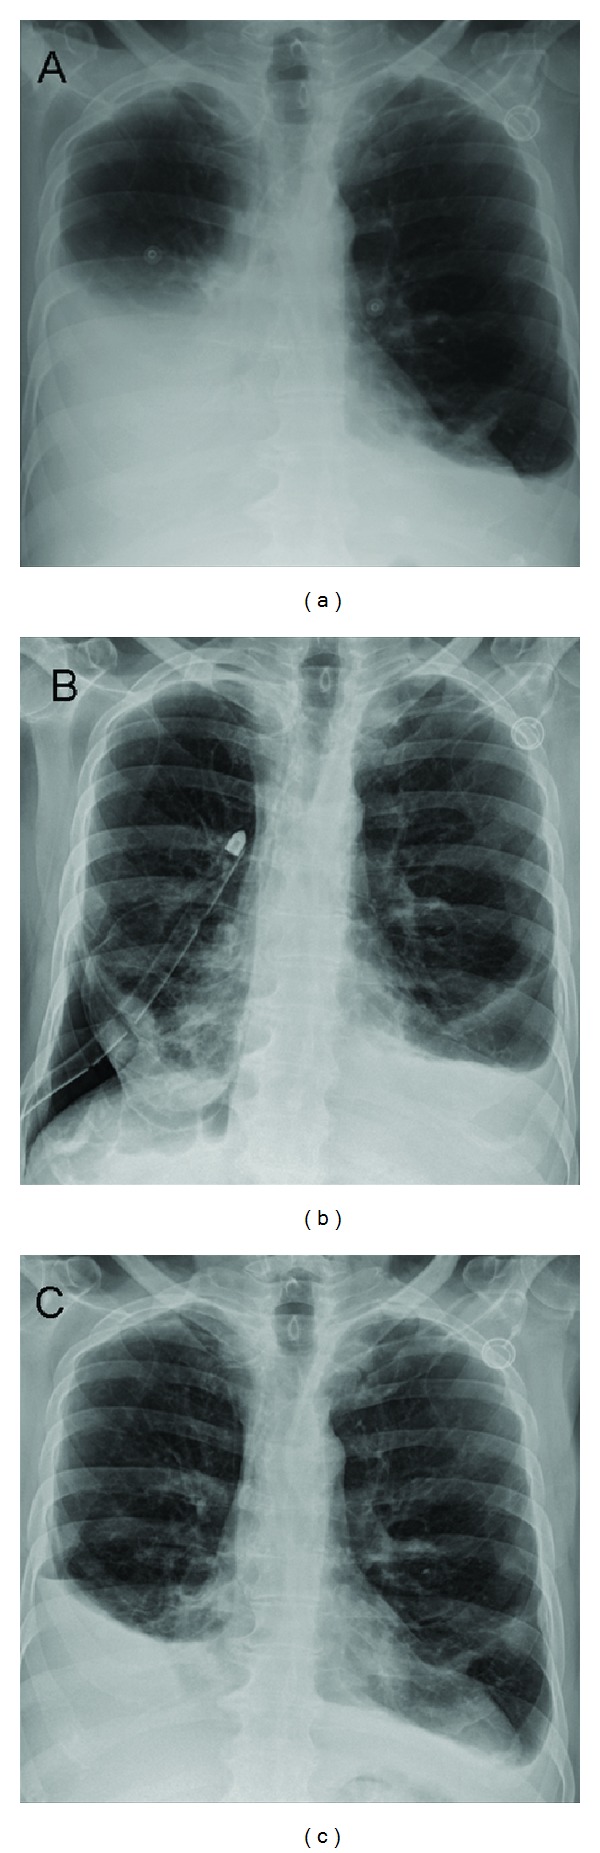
(a) Preoperative chest X-Ray, (b) chest X-Ray one day after thoracoscopic talc poudrage in a patient who received an unsuccessful pleurodesis, and (c) chest X-Ray 30 days after SNS slurry. (Images a, b, and c are from the same patient who received a repeated administration of SNS.)
